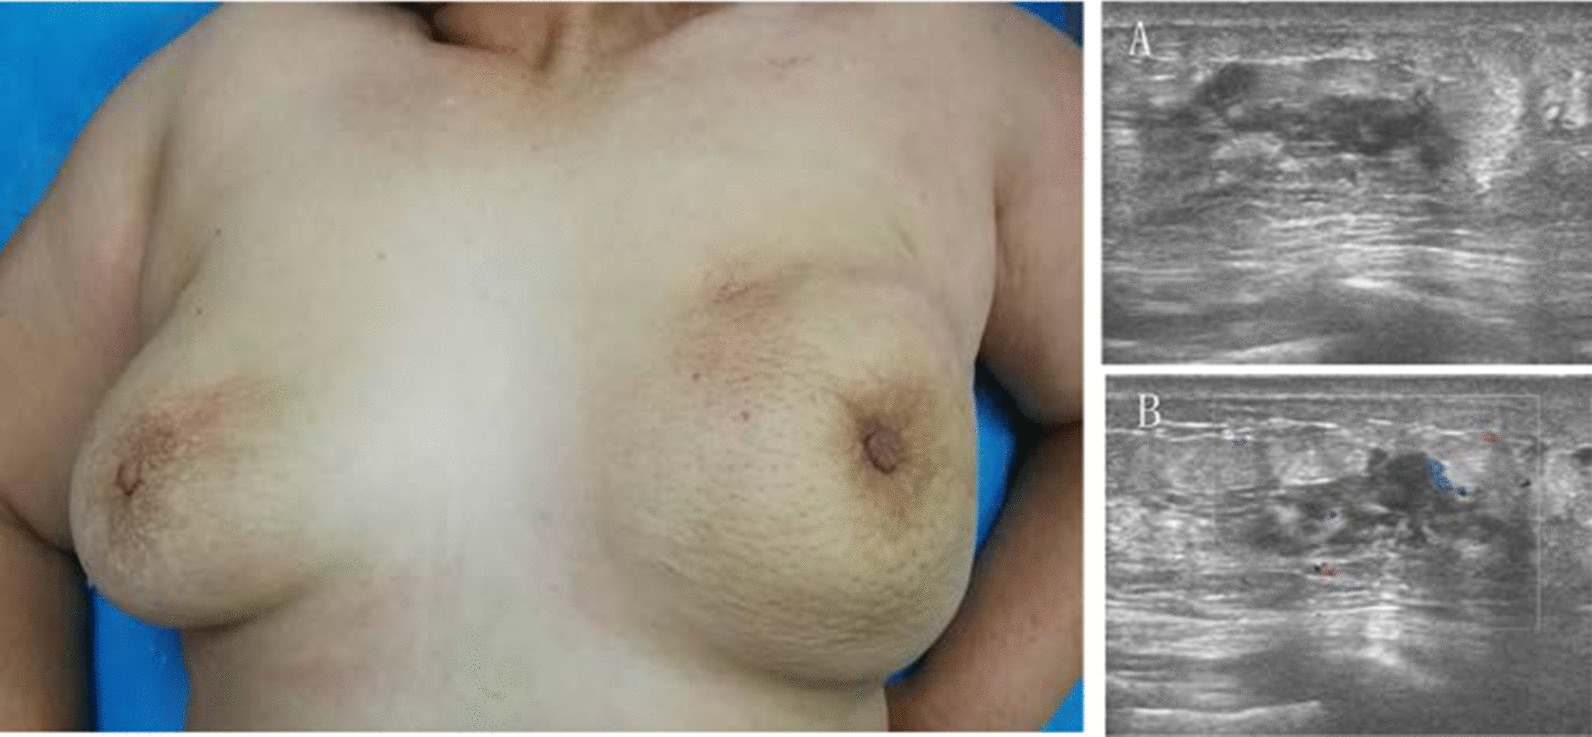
Gross finding and ultrasound (US) examination. Diffuse erythema, skin ridging and peau d’orange appearance of the left breast skin. a US showed a hypoechoic area with irregular shape and unclear border. b Color Doppler US showed increased blood flow within the lesion.
